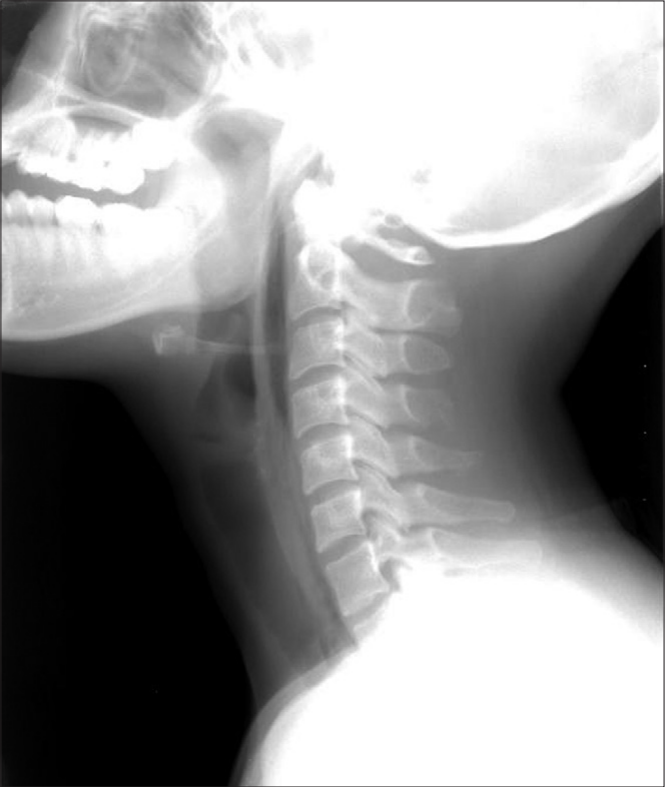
Lateral neck X-ray of case 2 reveals emphysema in the prevertebral space
